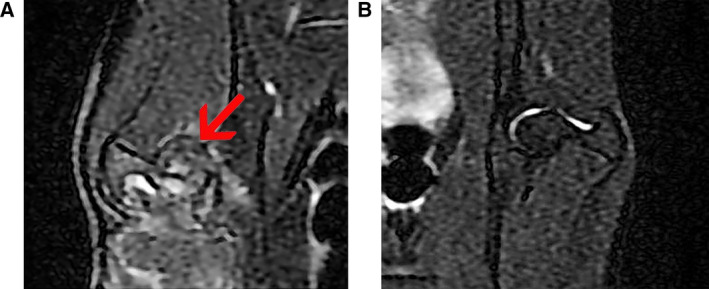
MRI examination of femoral head. (A) MRI of TIONFH. (B) MRI of normal femoral head. The crescents and collapses are indicated by the red arrow.
